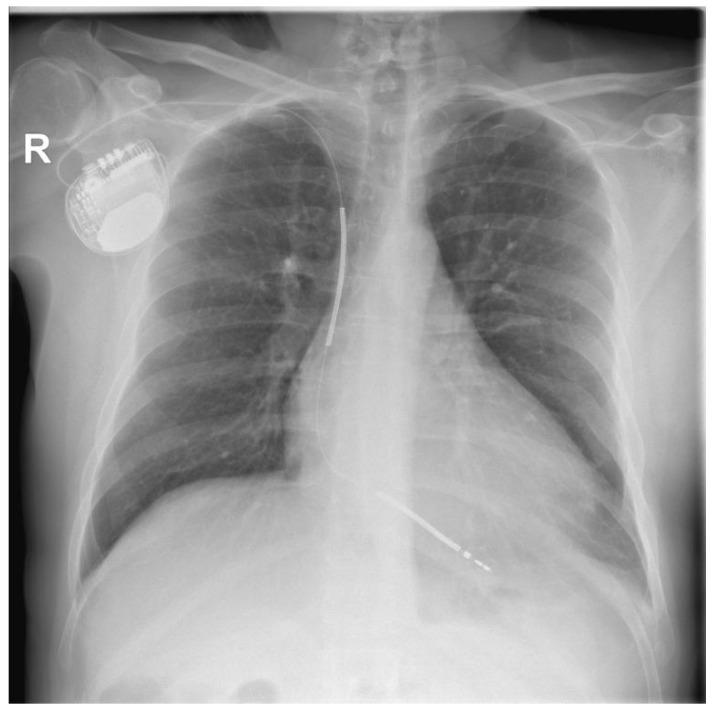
Chest X-ray with new defibrillator on the right side of the chest and the lead implanted through the right subclavian vein.
